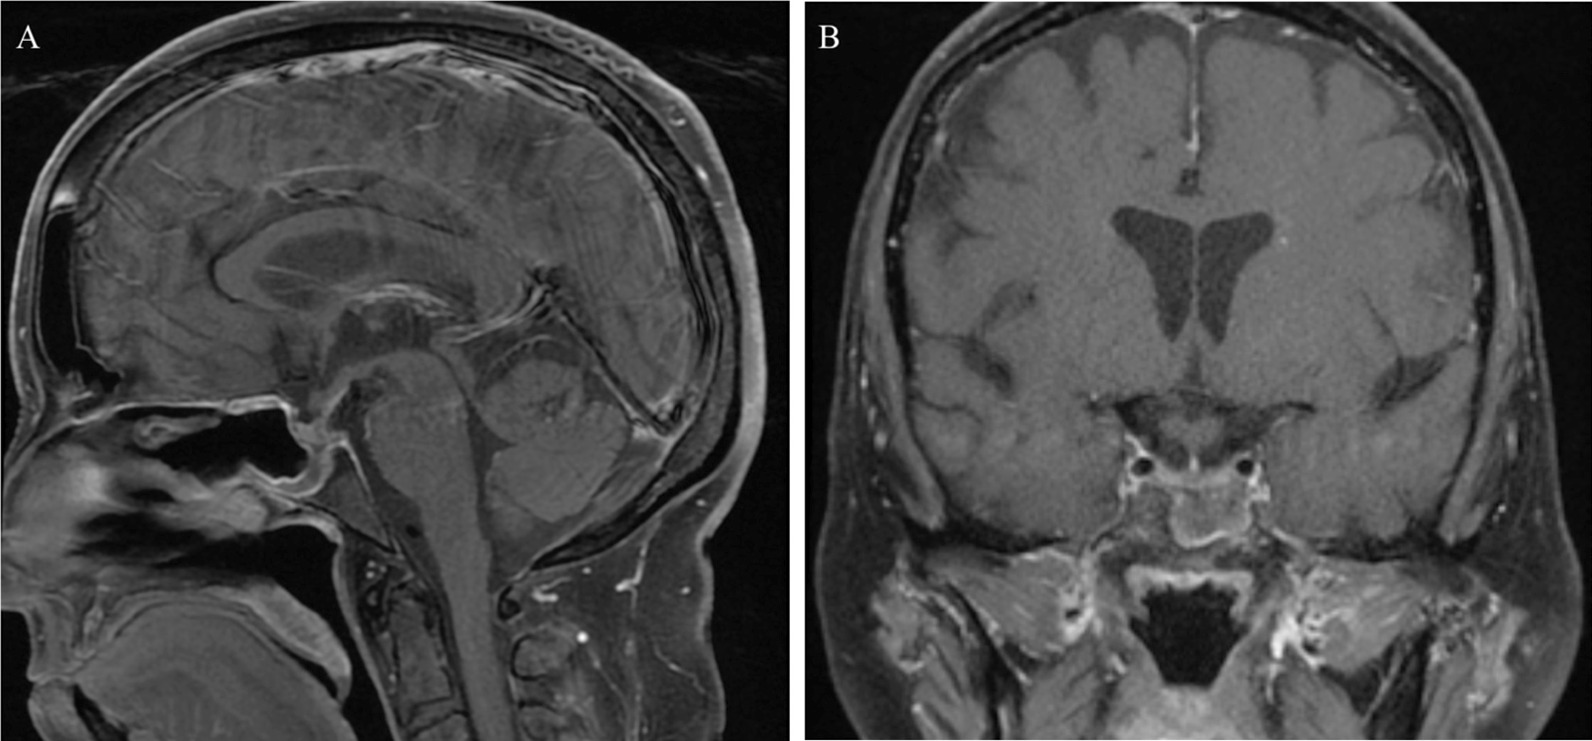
MRI brain with and without gadolinium 3 months post-biopsy showing complete resolution of abnormal enhancement and return to normal size of pituitary gland and stalk. Sagittal T1 post-gadolinium (A). Coronal T1 post-gadolinium (B)
